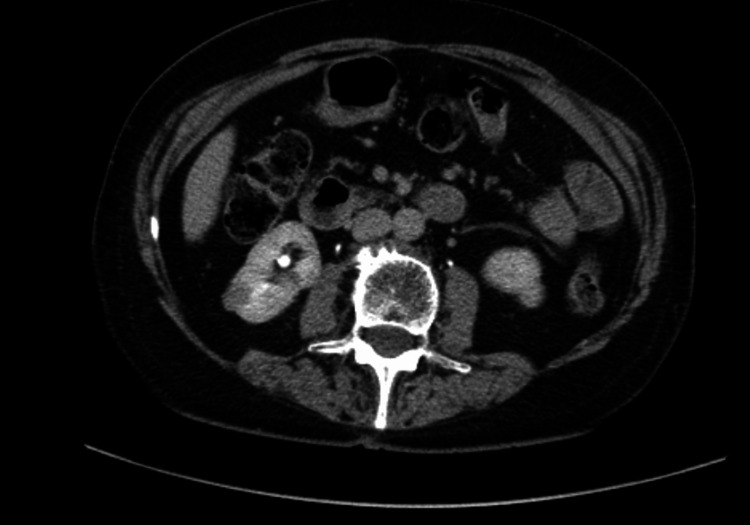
Interval axial CT abdomen 36 months after first imaging Interval axial CT demonstrating an increase in size of the renal lesion.
